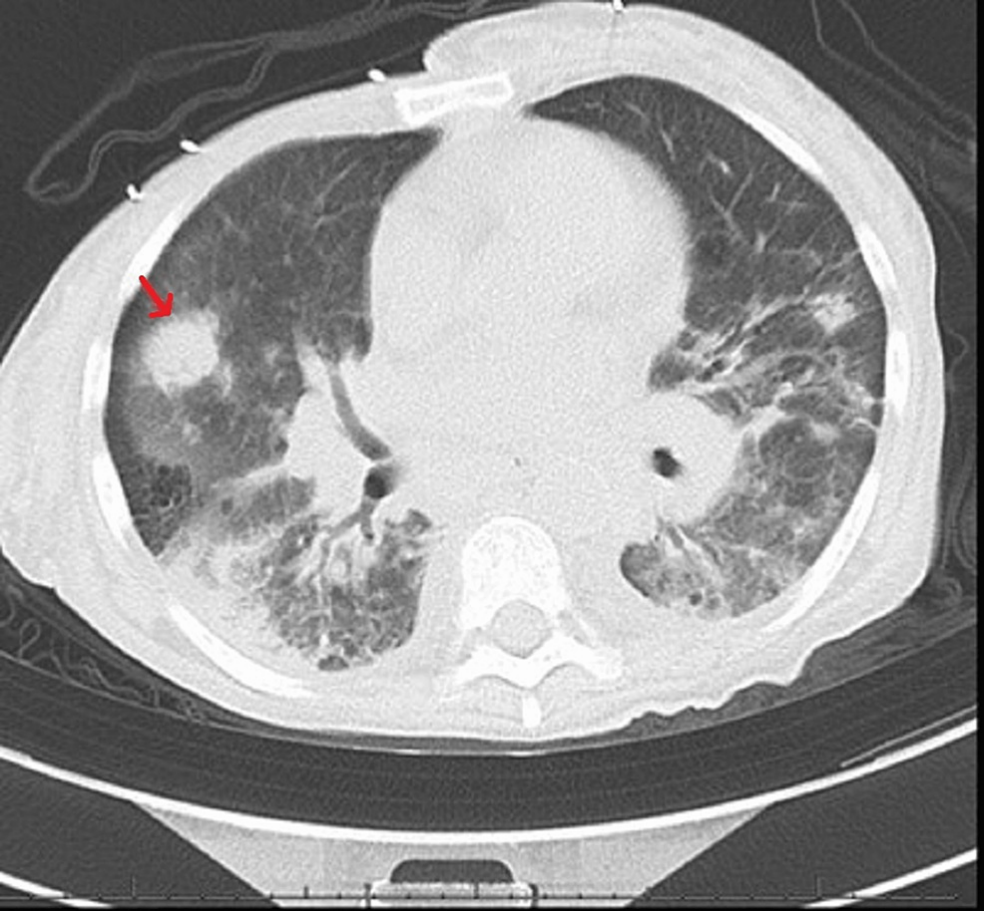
Computed tomography of the chest (axial view) showing a pulmonary nodule in the right middle lobe (red arrow) and bibasilar fibrotic changes.
